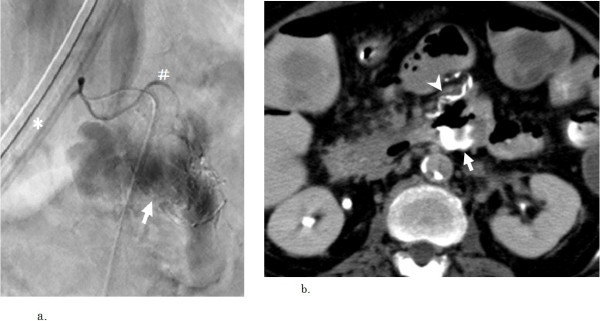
The final angiography and CT after embolization.
